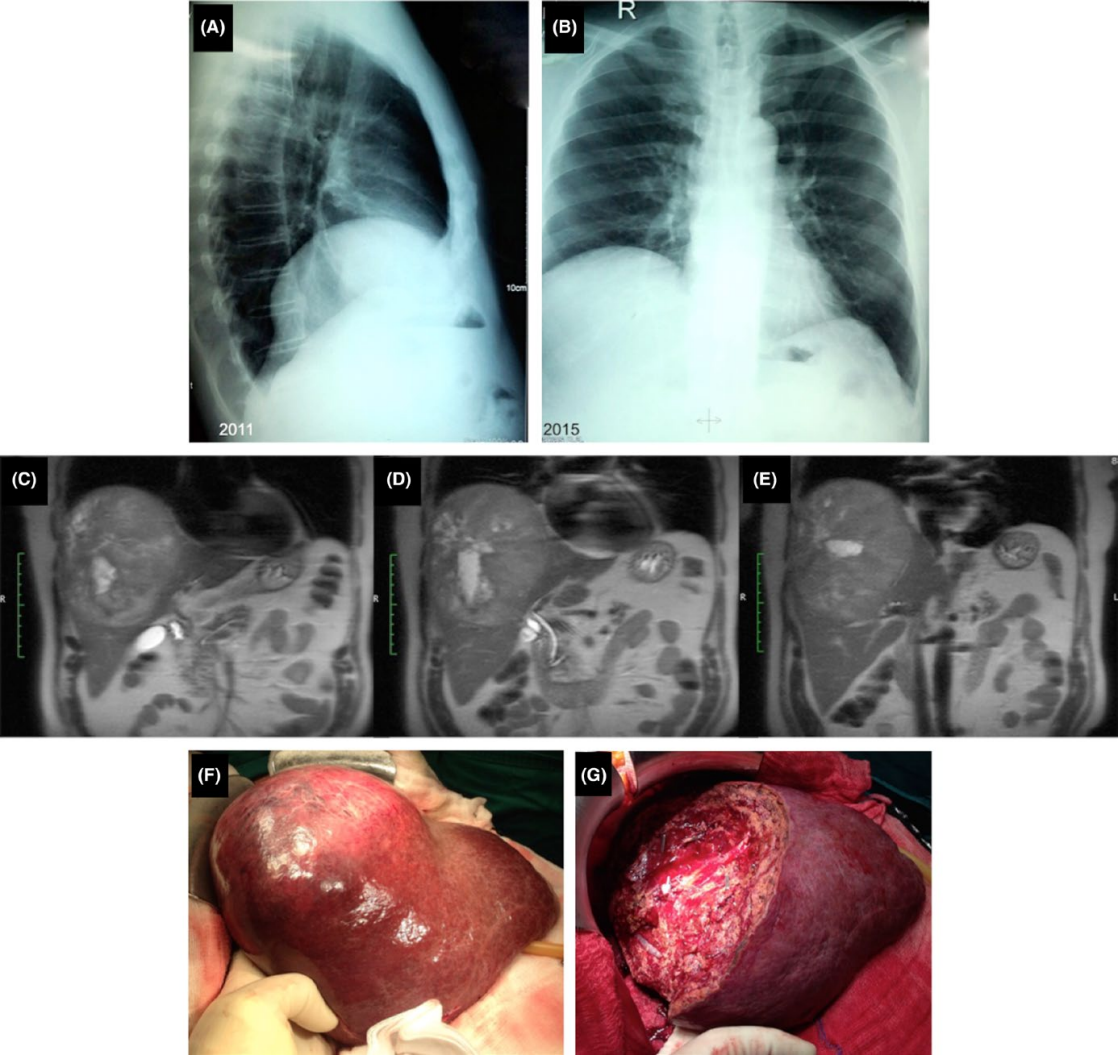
A, Previous thorax X‐ray of the patient (2011). B, Thorax X‐ray of the patient at presentation (2015). C‐E,Magnetic resonance imaging of the giant liver lesion. F, G, Intraoperative images of the central liver lesion prior (F) and after resection (G)
